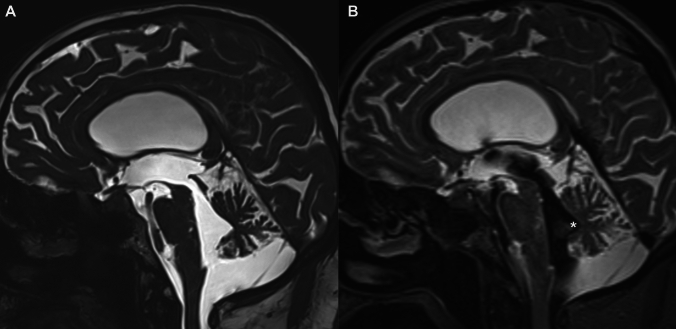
AB Sagittal T2-weighted CISS (A) and SPACE images (B) in a patient with iNPH. Note the presence of ventriculomegaly in lateral ventricles and hyperdynamic CSF flow in the fourth ventricle associated with marked signal void. Hyperdynamic CSF flow is also visible in the median dorsal recess (white asterisk)
